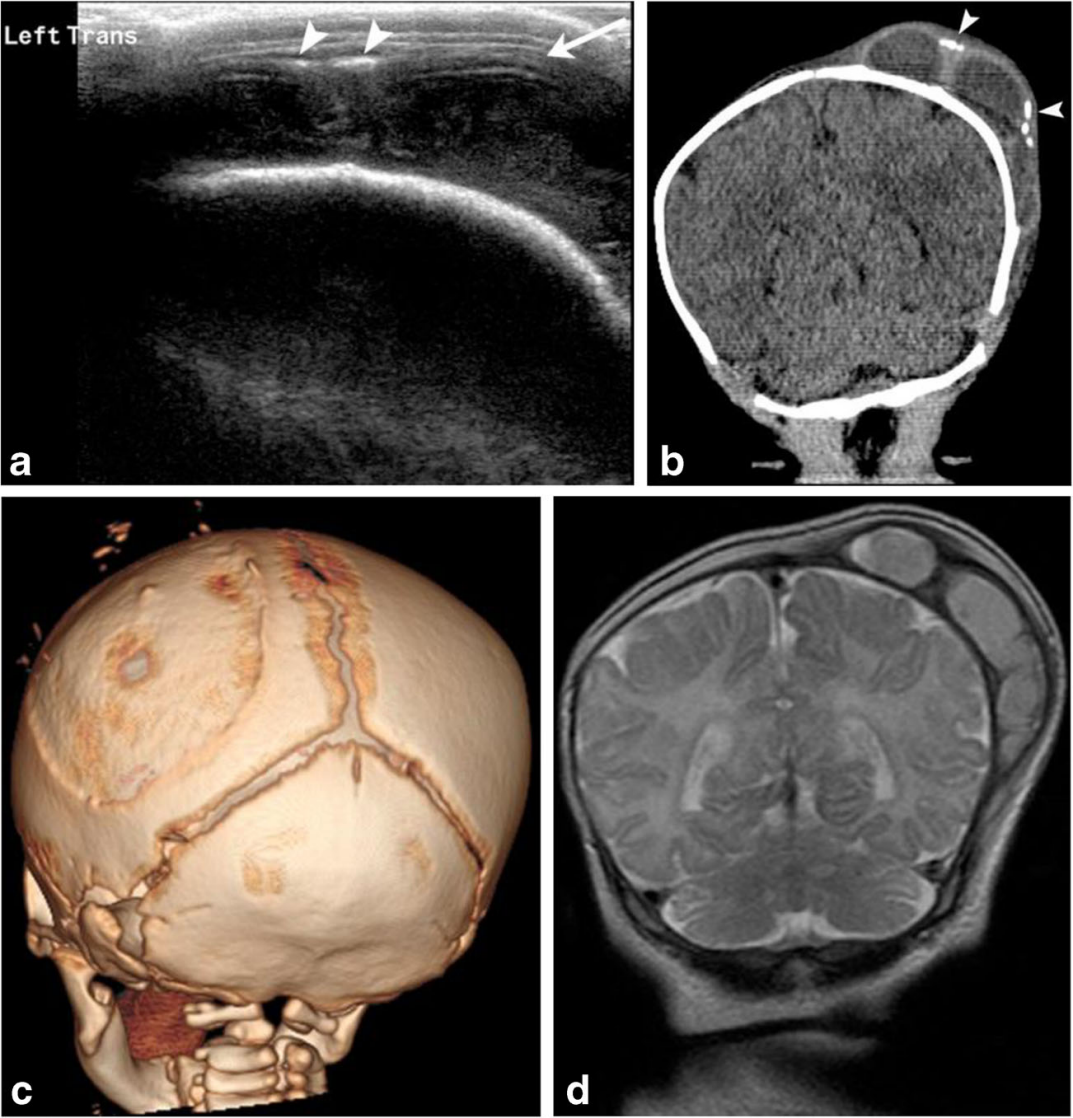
Cephalhematoma 1-month-old male with history of traumatic delivery presenting with right parietooccipital soft tissue swelling. Transverse grayscale ultrasound (a) image of the left parietooccipital scalp shows a complex fluid collection (arrow), with punctate linear echogenic foci along the superficial aspect (arrowheads), suggestive of calcifications. Relationship with the adjacent left lambdoid suture was difficult to evaluate by ultrasound. B. Coronal non-contrast head CT (b) image demonstrates a lobulated fluid collection with thick septations and peripheral calcifications (arrowheads) that does not cross the adjacent sagittal or the lambdoid suture, suggestive of cephalhematoma. 3-D volume (c) rendered images in bone algorithm shows cortical irregularity along the left parietal bone at the site of cephalhematoma as well as peripheral calcifications along the superficial aspect of the cephalhematoma. Coronal T2 image (d) from an MR exam obtained one week later in the setting of patient’s seizures re-demonstrates the large subperiosteal complex fluid collection with thick septations and isointense fluid signal consistent with evolving blood products in the known left parietal cephalhematoma
